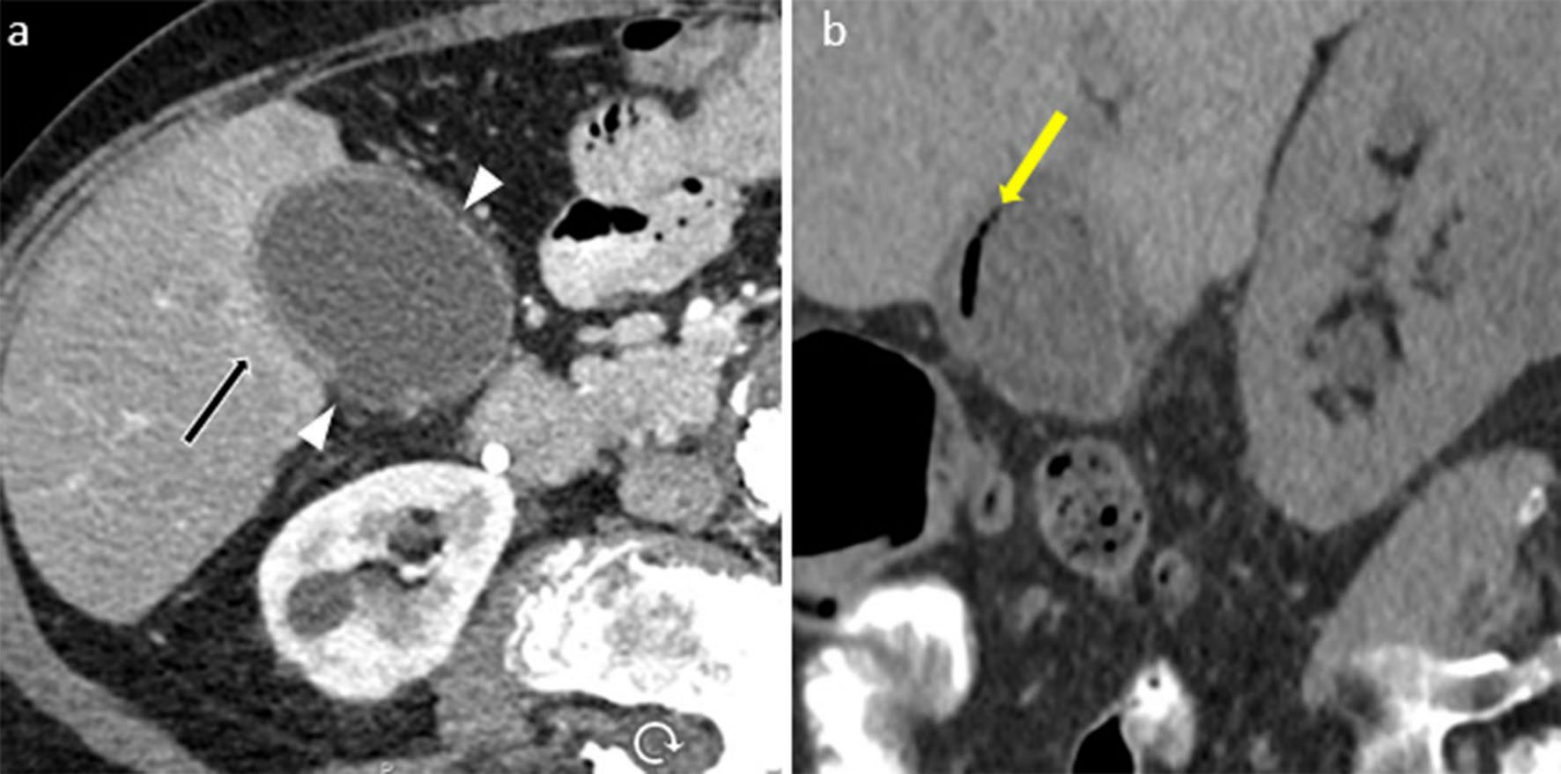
Gangrenous cholecystitis in a 68-year-old female with fever and diffuse abdominal pain post sigmoidectomy and colostomy. Contrast enhanced CT axial views (image a) demonstrates wall thickening and focal areas of poor or lack of gallbladder enhancement (arrowheads) with pericholecystic stranding, and increased enhancement of the adjacent liver parenchyma (arrow). The findings were complicated by development of gas in the wall (image b, yellow arrow) after 7 days of gangrenous cholecystitis diagnosis
